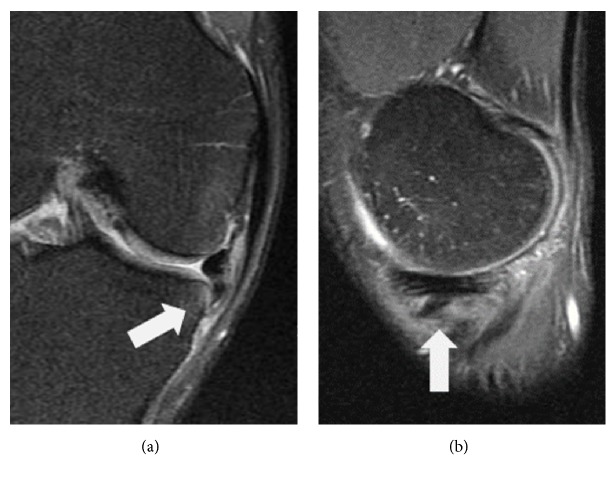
MRI of a 33-year-old male patient with an incarcerated inferiorly displaced flap tear of medial meniscus. (a) A coronal T2-weighted image and (b) a sagittal T2-weighted image of the most medial aspect of the knee showed that the displaced fragment (arrow) was located inferomedial to the tibial plateau and extended deep between tibia and medial collateral ligament.
